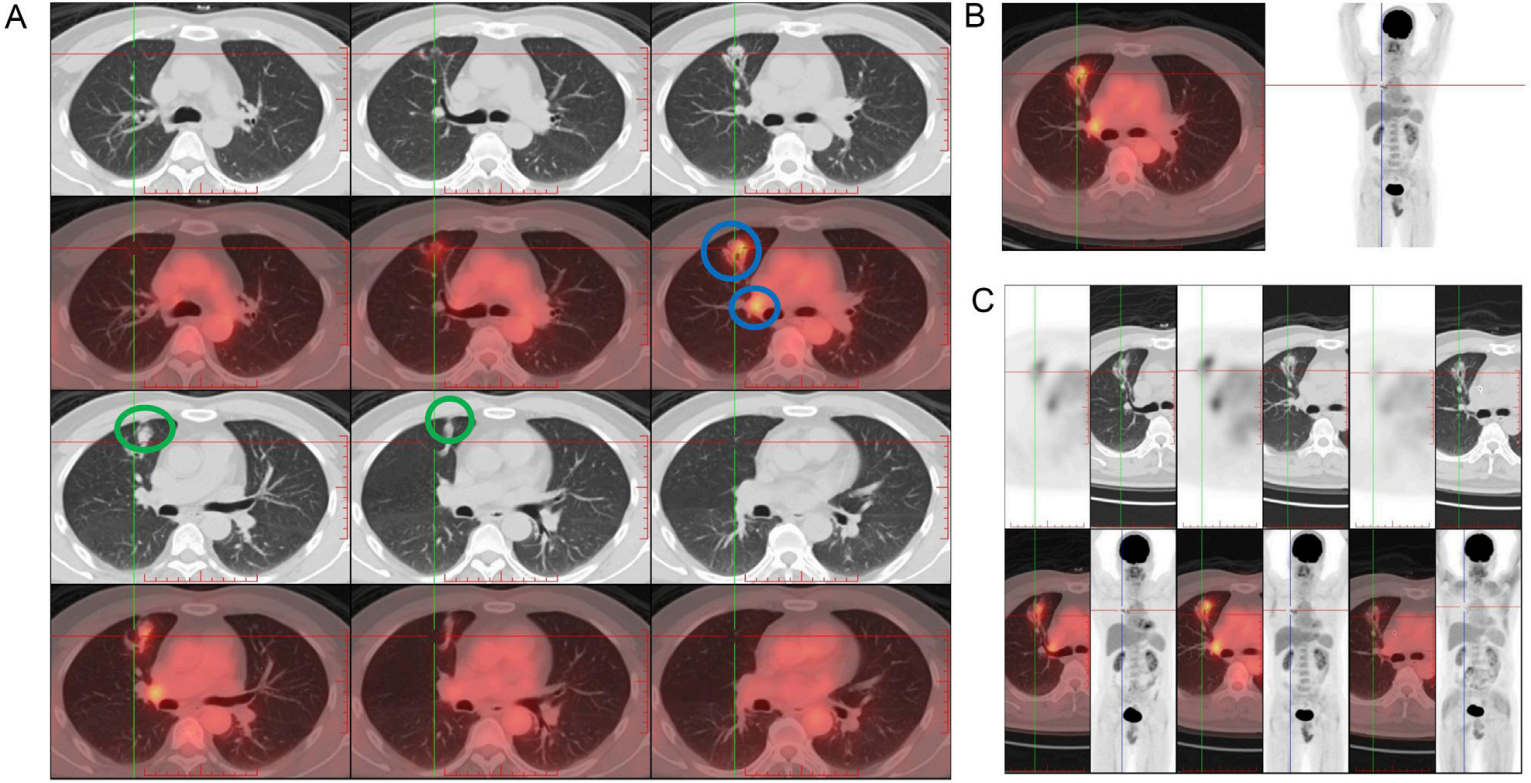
Initial imaging findings at diagnosis in January 2022. (A) Positron emission tomography-computed tomography (PET-CT) scan at the time of diagnosis in January 2022 revealed an irregular mass and involved lymph node (blue circles) in the anterior segment of the right upper lobe, raising suspicion of malignancy and adhesions to the chest wall and the interlobar pleura (green circle). (B) PET-CT scan showed moderately elevated and heterogeneous metabolic activity of the lesion and mildly hypermetabolic right hilar lymph nodes consistent with nodal involvement (left panel) and potential adhesion of the lesion to the anterior chest wall and to the interlobar pleura along the right horizontal fissure, with tractional deformation of the interlobar pleura. The coronal view is shown in the right panel. (C) After two cycles of T-DXd treatment, PET-CT scan in April 2022 showed a significant reduction in the size of the right upper lobe lesion.
